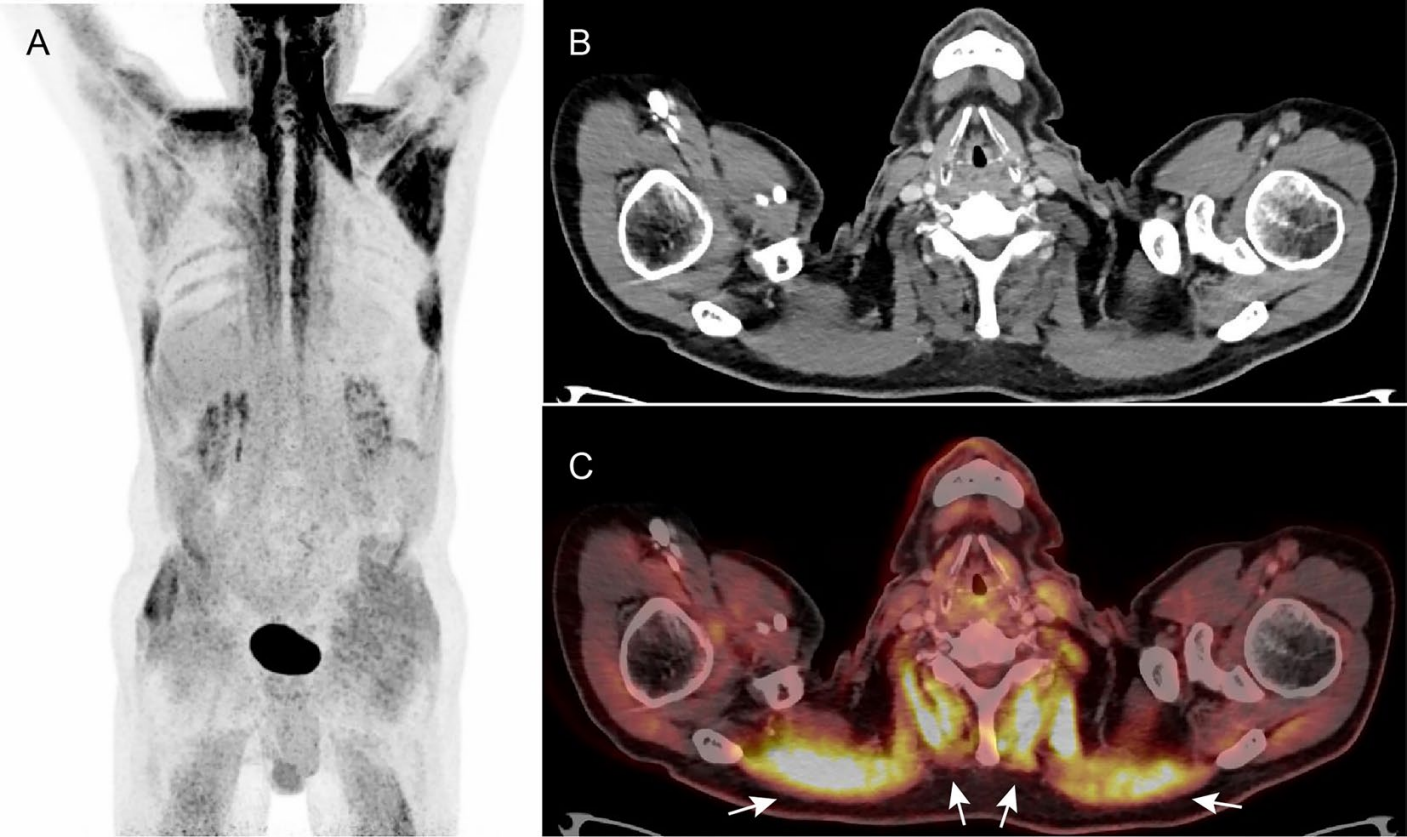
2‐[^18^F] FDG PET/CT scan of the patient before treatment. (A) Maximum intensity projection shows markedly high and symmetrical 2‐[^18^F] FDG uptake in the neck and shoulder muscles as well as high uptake in muscles of the gluteal region and both arms and legs. (B, C) CT and the fused PET/CT image demonstrates high 2‐[^18^F] FDG uptake in the neck muscles (arrows).
